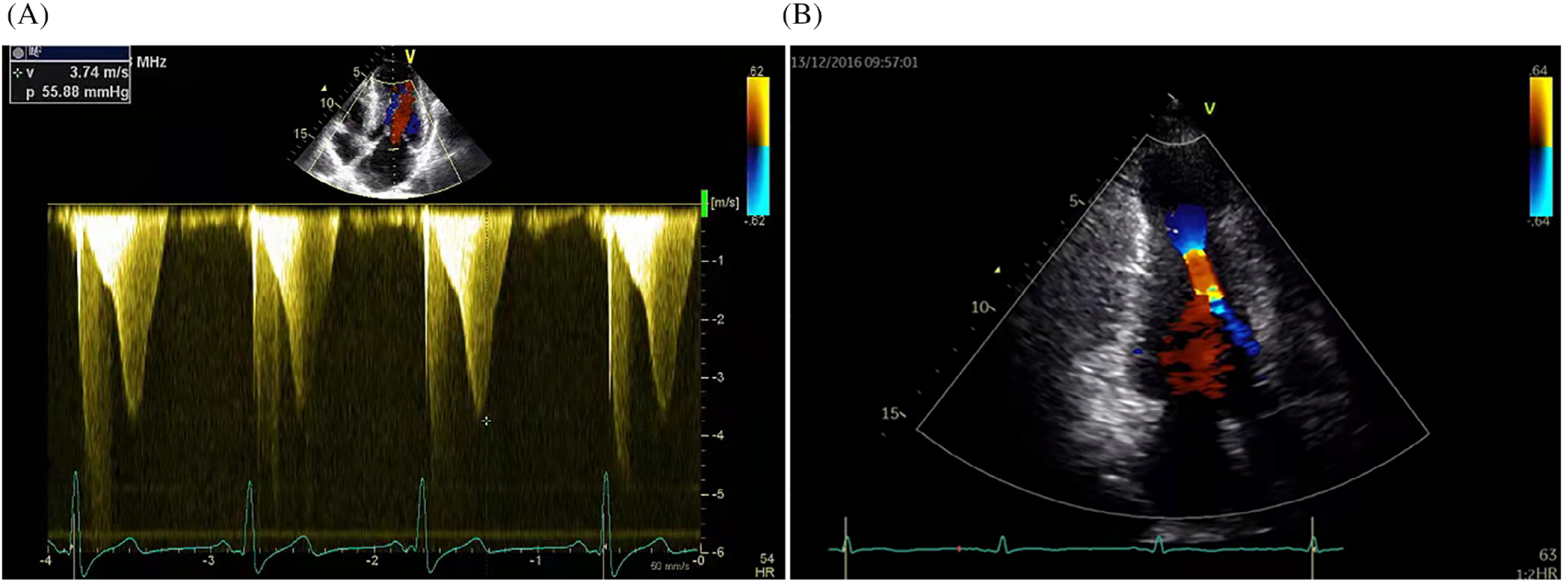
(A) A dagger‐shaped waveform is obtained, showing a high mid‐ventricular gradient, and the estimated peak velocity of 3.74 m/s. (B) Two‐dimensional transthoracic echocardiography from the LV long‐axis two‐chamber view showing an hourglass shape of the left ventricle during systole. LV, left ventricular
